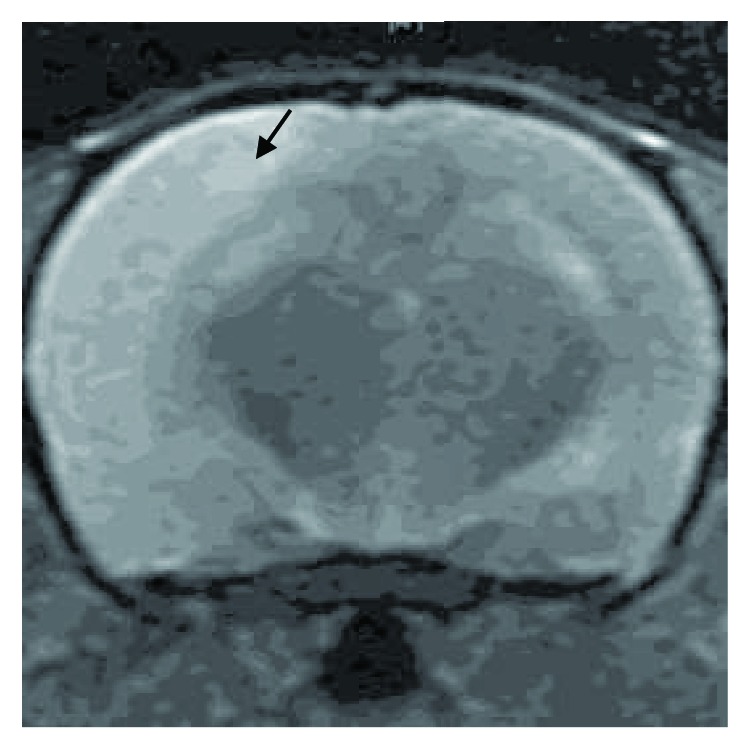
Infarct region performed T2 hyperintensity changes. T2-weighted magnetic resonance image revealed an abnormally increased T2 signal in the primary ischemic region (arrow).
